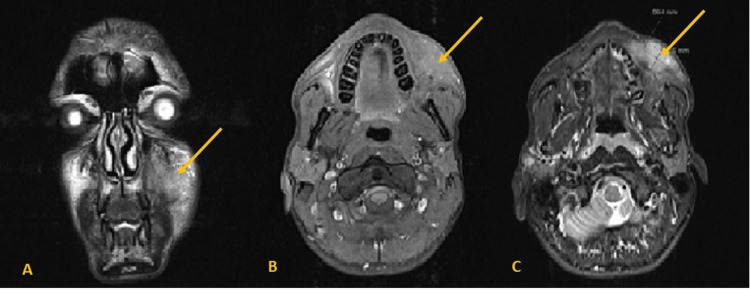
Face MRI Initial MRI of the face A: T2 weighting with fat saturation, B: T1 weighting after contrast, C: T2 weighting
